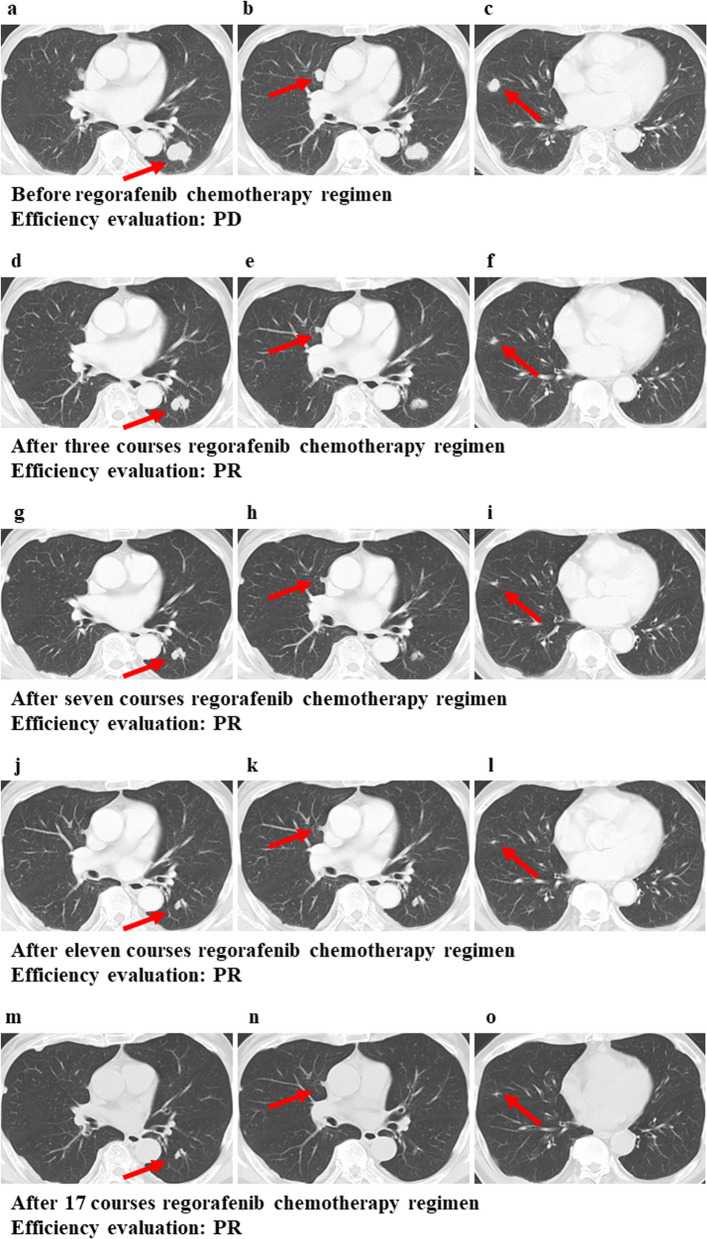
Consecutive CT findings from the start of third-line chemotherapy to the end of 17 courses. a–c The lung tumors had progressed after 11 courses of the FOLFIRI + RAM chemotherapy regimen had been administered. d–f The lung tumors had shrunk after three courses of the regorafenib chemotherapy regimen. g–i The lung tumors had shrunk after seven courses of the regorafenib chemotherapy regimen. j–l The lung tumors had shrunk after eleven courses of the regorafenib chemotherapy regimen. m–o The lung tumors had shrunk after 17 courses of the regorafenib chemotherapy regimen. These arrows indicate lung metastases
